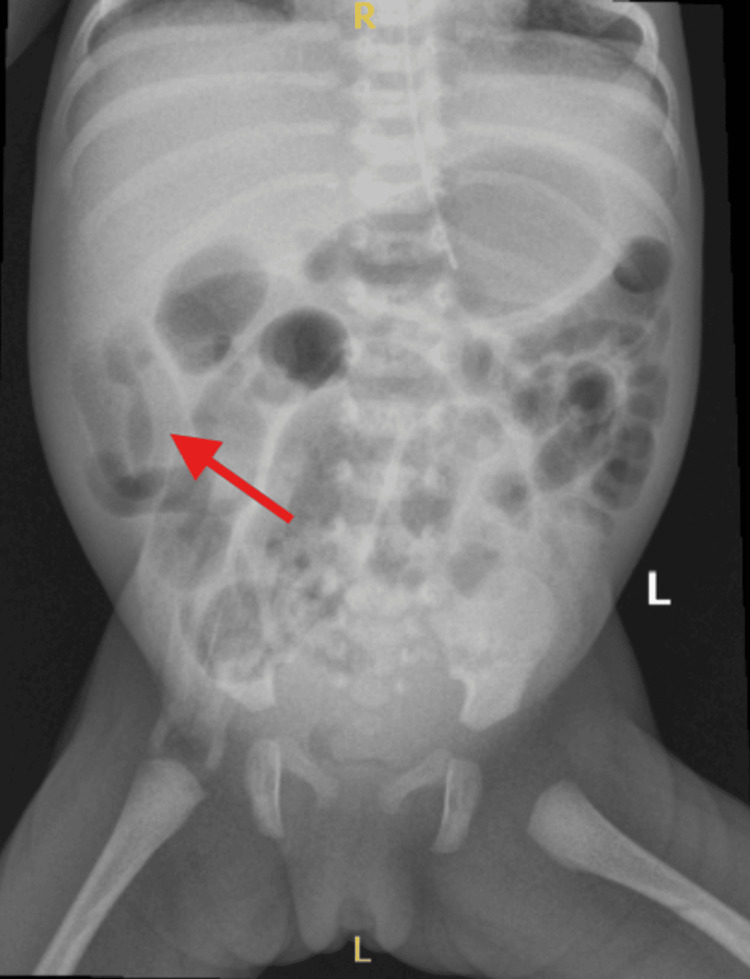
Case 1 - Abdominal X-ray showing dilated bowel loops and no signs of pneumatosis or free air underneath the diaphragm. The red arrow indicates dilated bowel loops.
